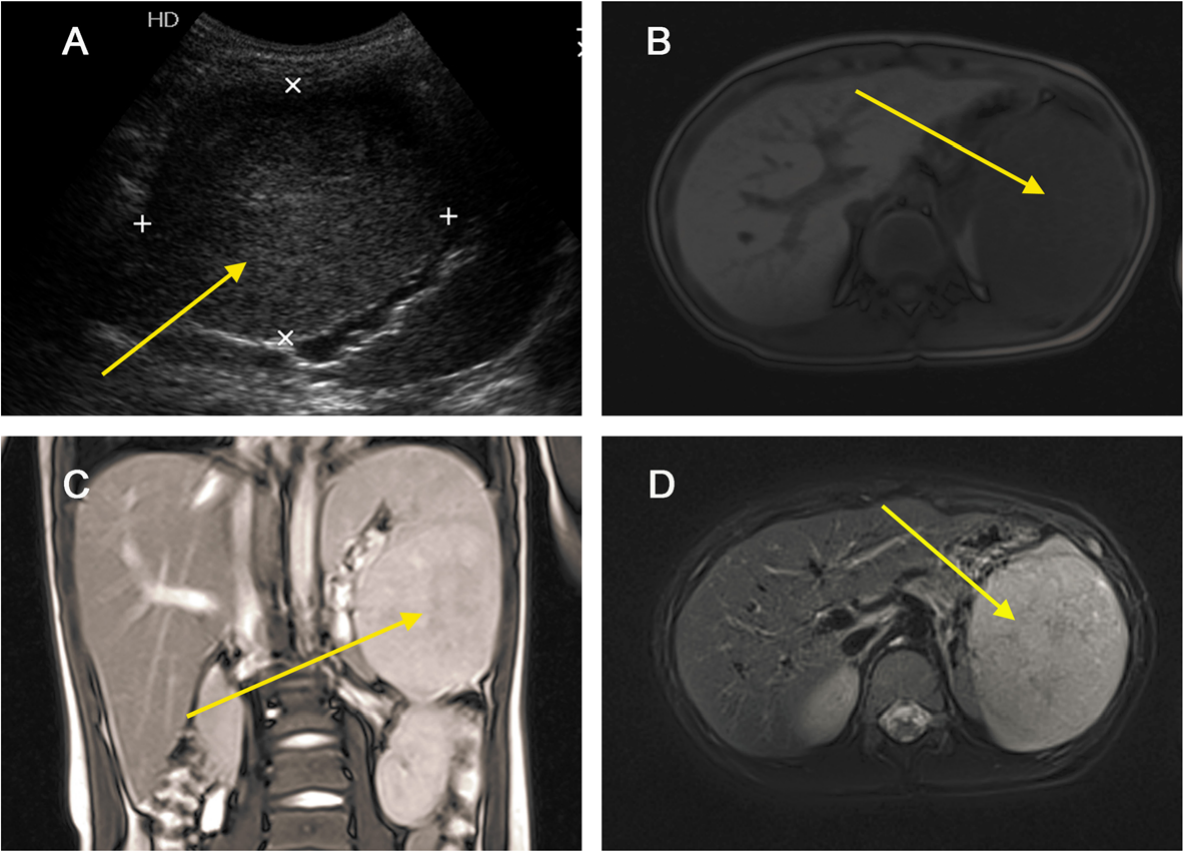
Splenic hamartoma in a 6-year-old boy with left upper quadrant abdominal pain. (A) Abdominal ultrasonography showed a hypoechoic mass (*arrow*) with well-defined borders in the lower pole of spleen. Magnetic resonance imaging (MRI) showed slightly hyperintense signal in the T1WI (B), T2WI images (C), and T2WI + Fatsat images (D), and a non-homogeneous signal within the lesion (*arrow*).
